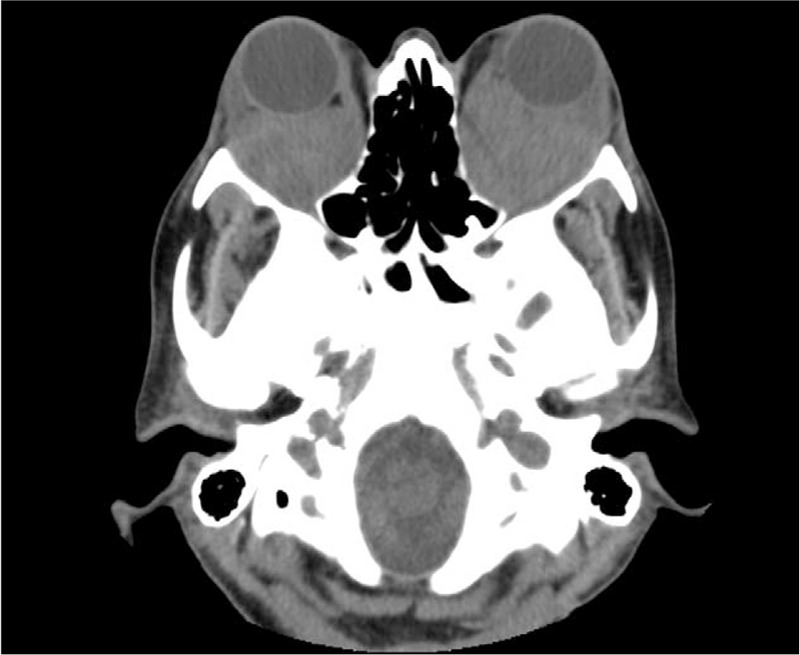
Axial cerebral computed tomography scan image of bilateral retro-orbital tissue causing exophthalmos.
